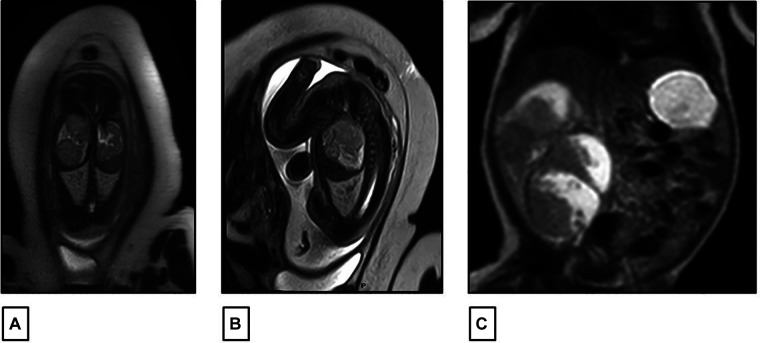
(A,B) Fetal MRI performed at 34 weeks of gestation showing a right renal fossa occupied by a 60 mm × 30 mm multinodular solid mass with intermediate signal intensity on T2. (C) Postnatal abdominal MRI showing a lobulated heterogeneous mass in the right renal fossa 81 mm × 58 mm.
